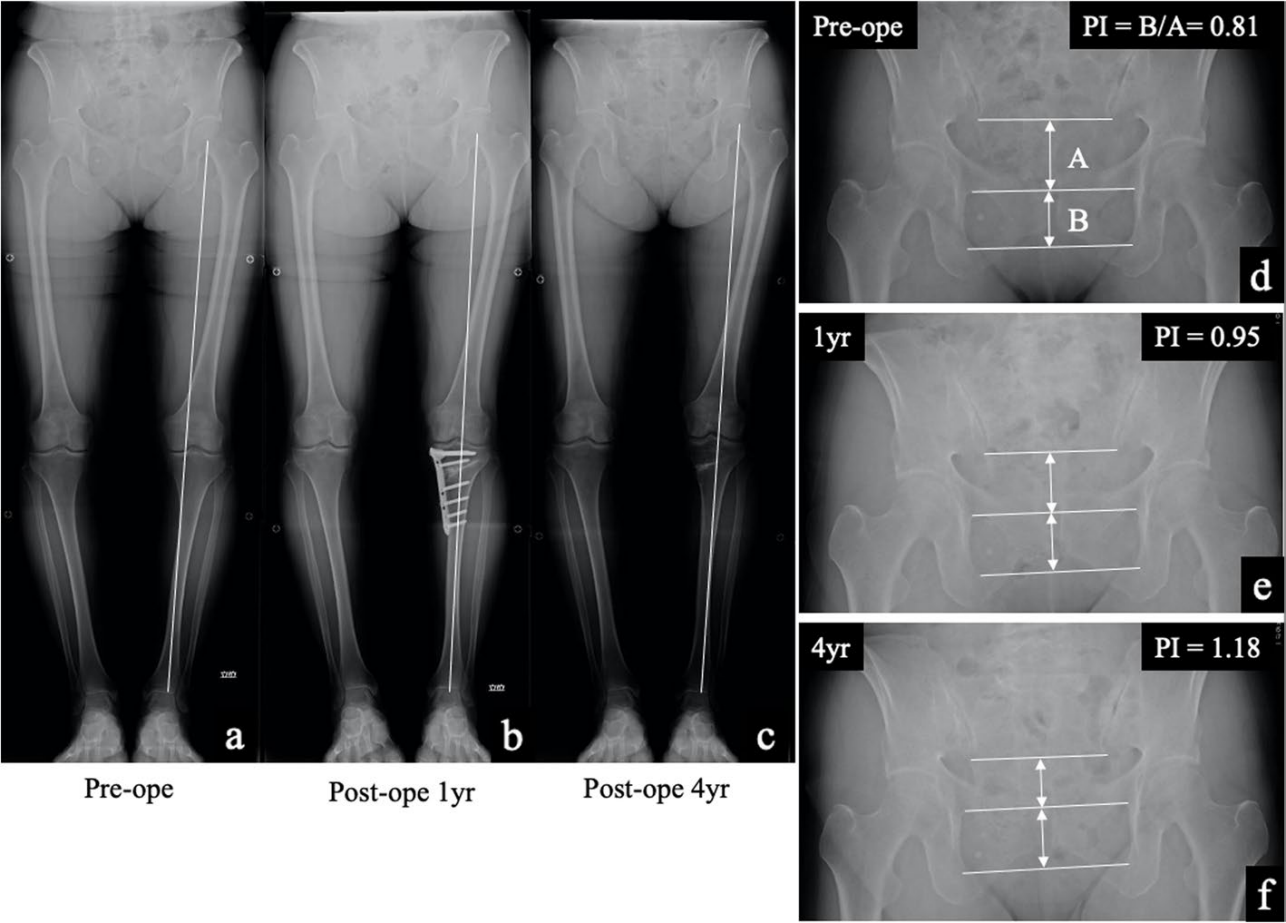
The pelvic inclination ratio. a-c Representative changes in pelvic inclination are shown. Representative whole-leg radiographs, in standing, are shown for a 78-year-old woman at the following time points: preoperatively and at 1 and 4 years after OWHTO, and at the final follow-up assessment. d-f Pre- to postoperative changes in the PI ratio are shown. The preoperative PI ratio of 0.81, 0.95 at 1 year after OWHTO and increased to 1.18 at the final follow-up
